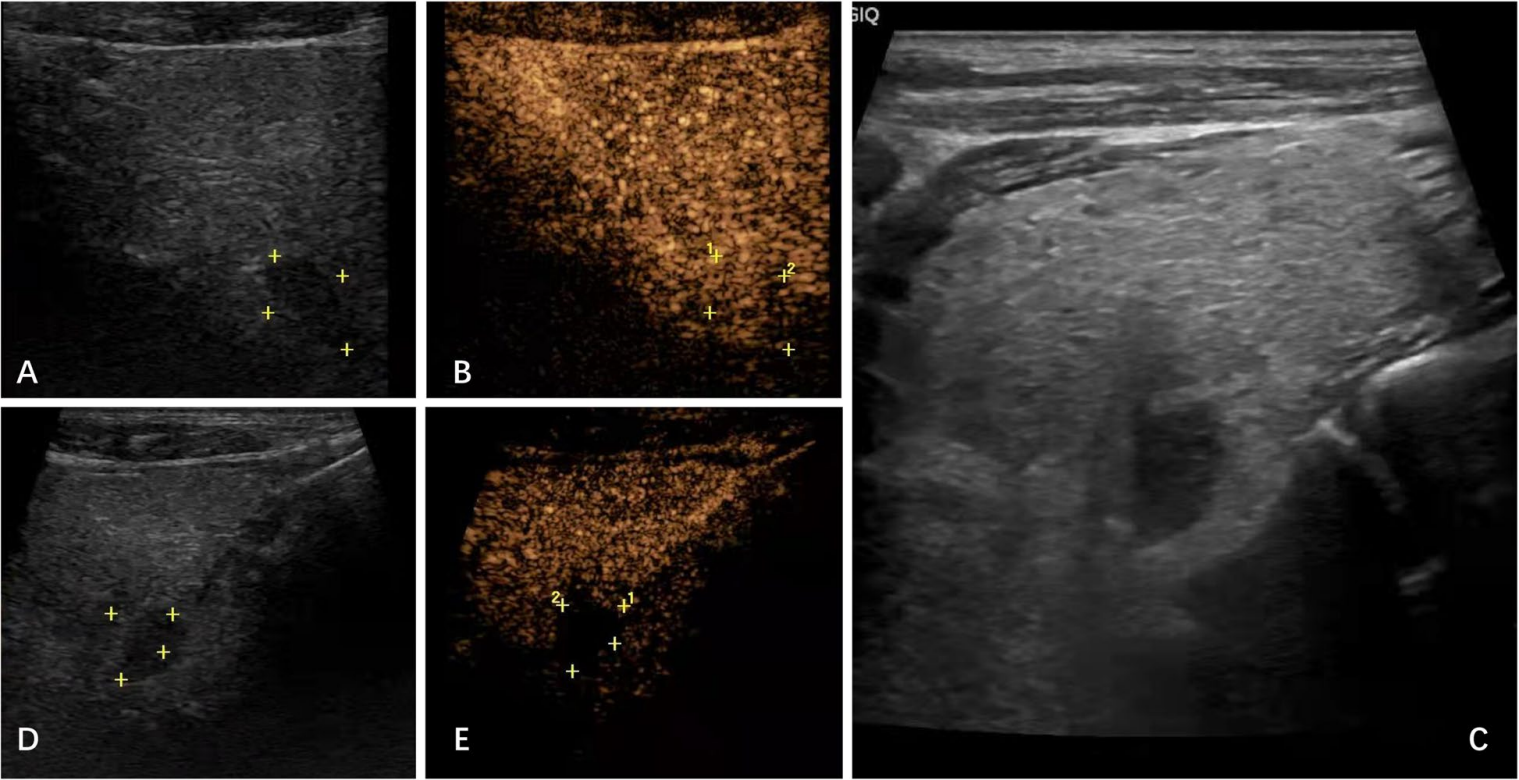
A Gray scale ultrasonography of neck before ablation (Color box placed in the suspected parathyroidoma lesion); B Neck CEUS before ablation showed continuous enhanced lesion (1.4cmx1.0cmx1.0 cm); C Ultrasonography visualization during ablation; D Neck Gray scale ultrasonography visualization after ablation; E Neck CEUS after ablation
